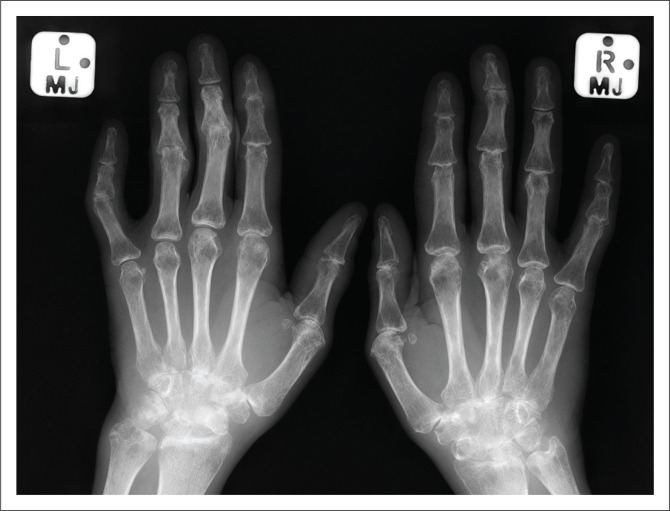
Frontal radiographs of both hands in an HIV-positive patient presenting with polyarthritis. RF was negative. Radiographs demonstrate bilateral severe peri-articular osteopaenia, severe joint space narrowing involving almost all joints and subluxation at the 3rd, 4th and 5th proximal interphalangeal joints.
